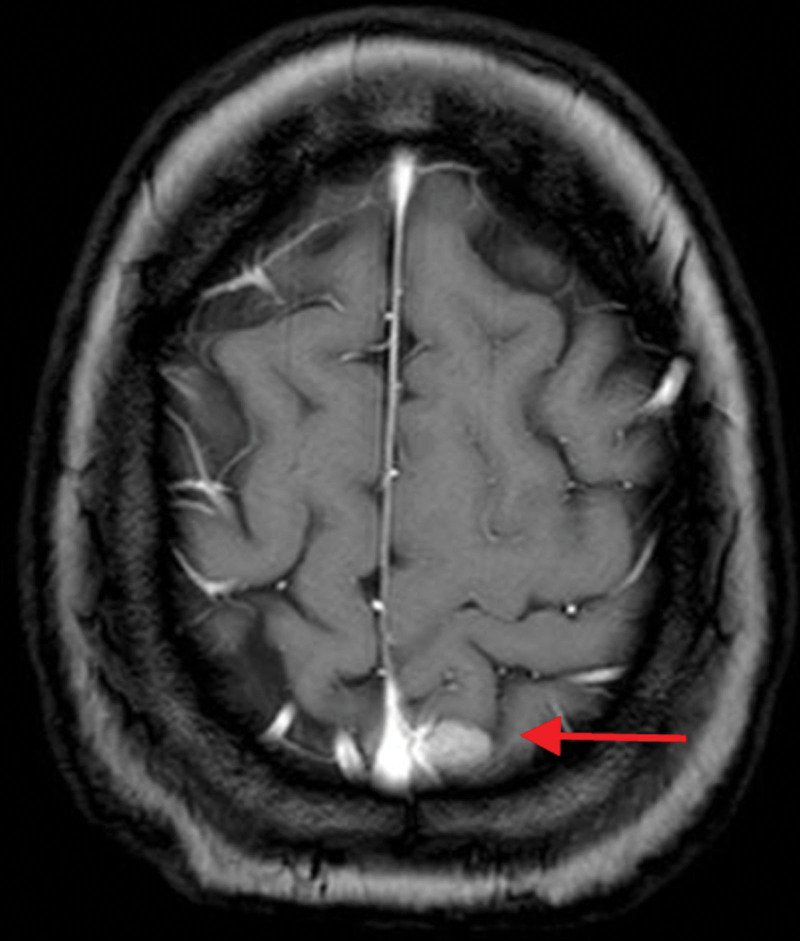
Head enhanced MRI reexamination showing a weakened enhancement of the nodule and no significant change in size (nearly 2 months after SRS). MRI = magnetic resonance imaging, SRS = stereotactic radiosurgery.
